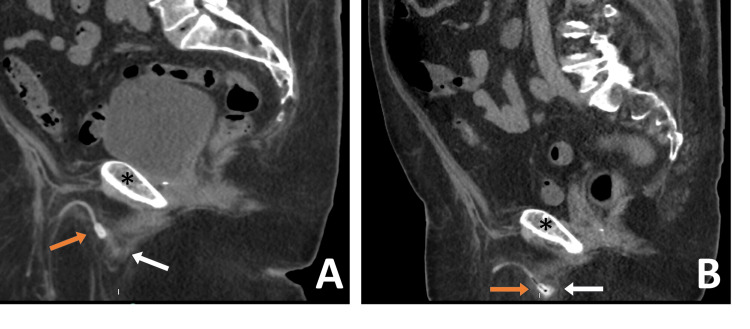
Sagittal CT scans. (A) Prior to surgical revision, the notch of the AUS tubing hiatus (orange arrow) can be seen to be misaligned with the urethra (white arrow) and at the level of the pubic bone (black asterisk); (B) Study performed for unrelated indication two months following surgical revision demonstrates the notch of the AUS tubing hiatus (orange arrow) is positioned in line with the urethra (white arrow) and inferior to the pubic bone (black asterisk). AUS: artificial urinary sphincter
